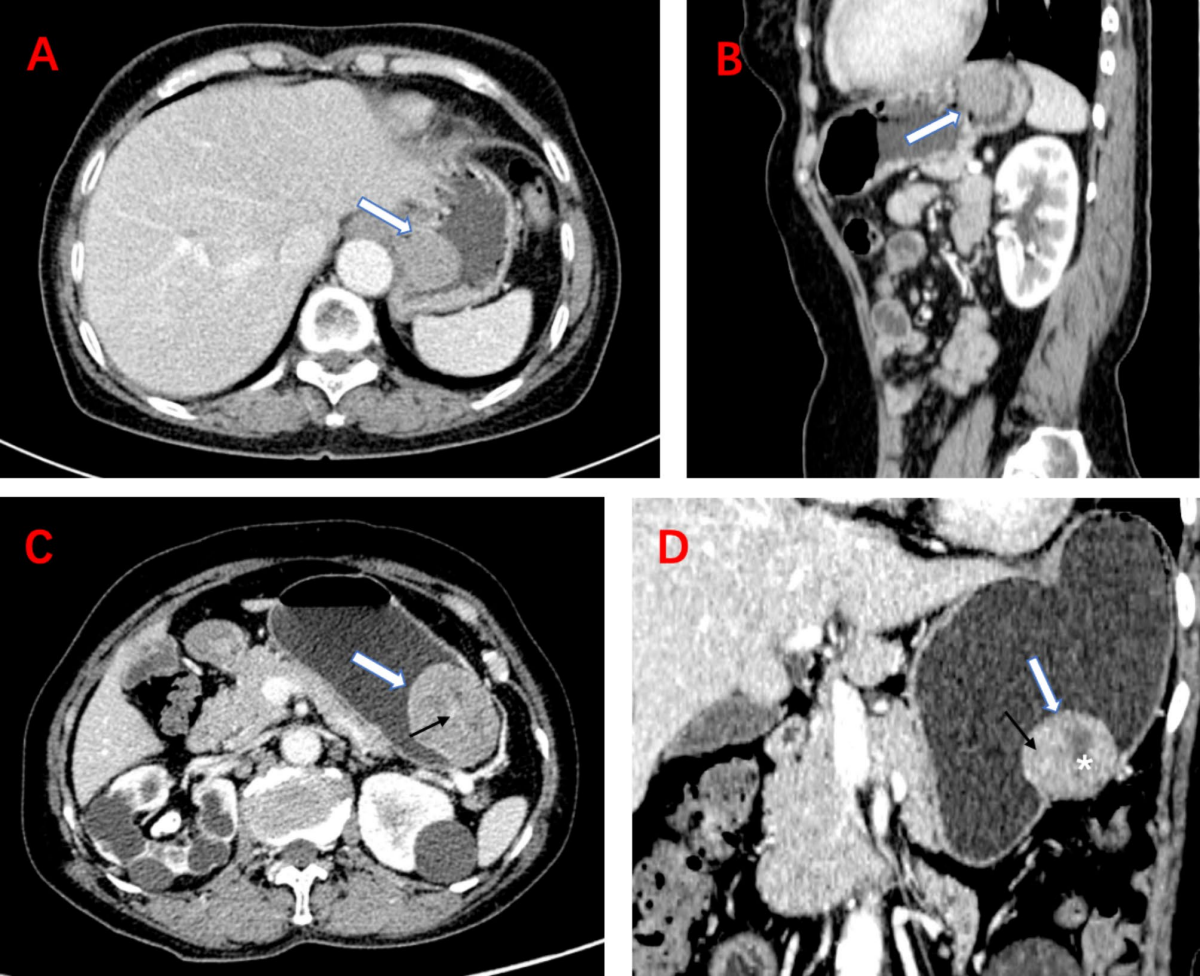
CT image of low-grade and high-grade malignant GISTs. (A-B). A low-grade malignant GIST in cardia in an elderly man. (A-B). Axial and sagittal CT scans in portal venous phase show a round mass with 2.5 cm of the long diameter (white arrows). The lesion has a homogeneous enhancement pattern without necrosis, calcification, and intratumoral angiogenesis in tumor. (C-D). A high-grade malignant GIST in gastric body in a middle-aged woman. Axial and coronal CT images in portal venous phase show an irregular neoplasm with 4.7 cm of the long diameter (white arrows). Intratumoral angiogenesis (black arrows) are seen in the lesion and the mass shows heterogeneous enhancement with necrotic portion (*) within the tumor
